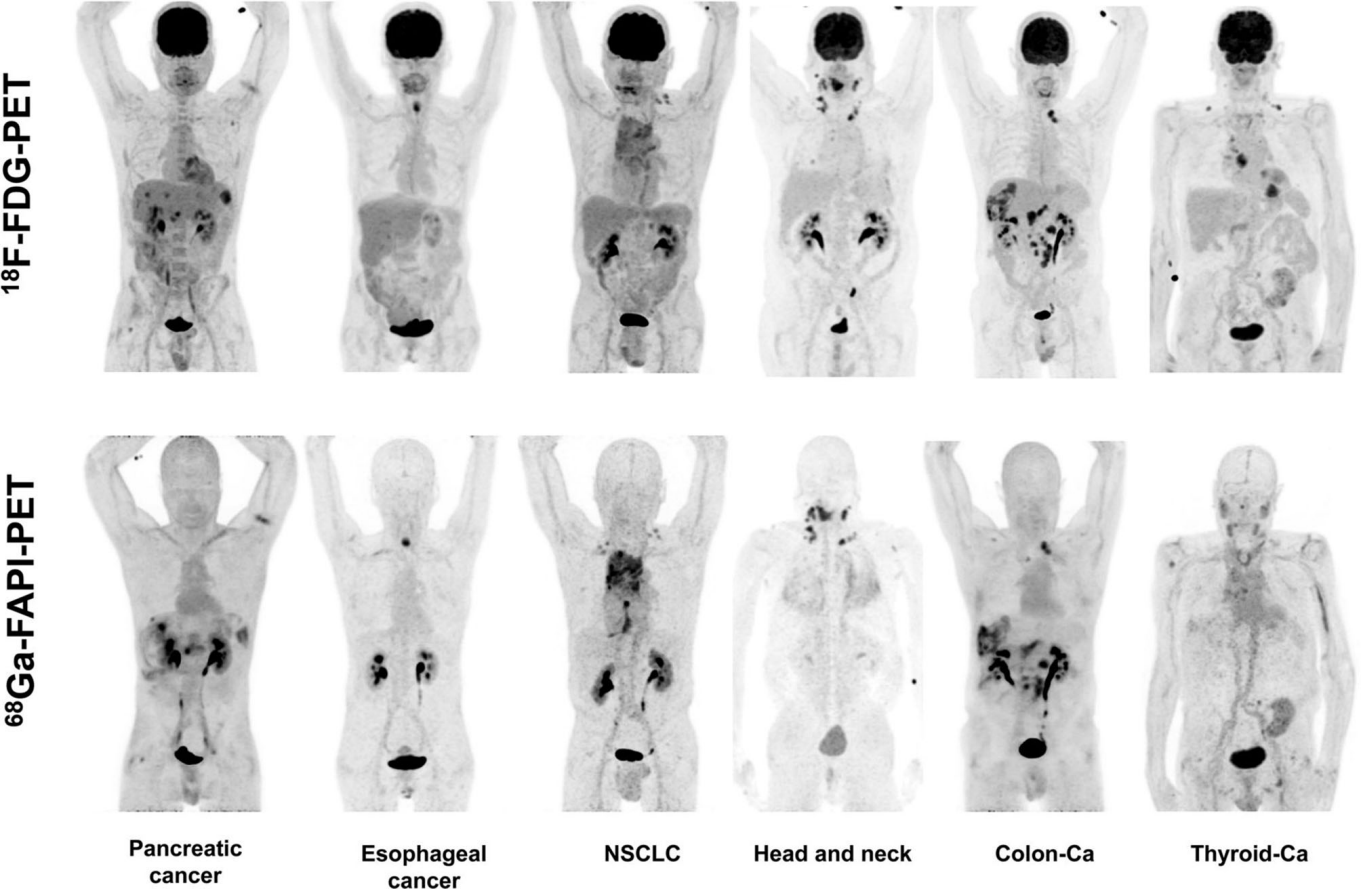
Six patients underwent intra-individual comparison of ^18^F-FDG-PET and ^68^Ga-FAPI-PET imaging over a 9-day period. Five of the six patients showed similar high tumor uptake in ^18^F-FDG-PET and ^68^Ga-FAPI-PET, and three of the six patients had more lesions detected due to low background uptake in the visceral or pharyngeal mucosa. Patients with iodine uptake negative thyroid cancer exhibited low ^68^Ga-FAPI tracer uptake compared with ^18^F-FDG-PET; Ca, cancer; NSCLC, non–small cell lung cancer (31).
